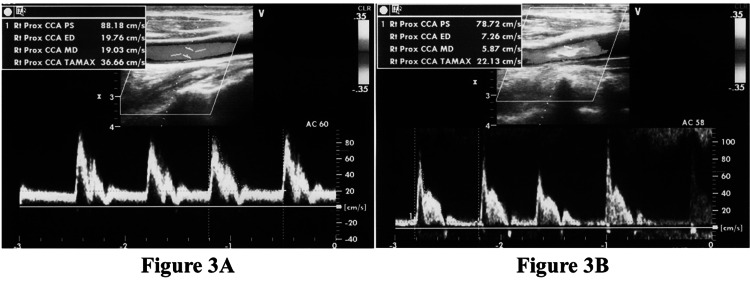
Ultrasound evaluation of a 73-year-old man with left hemiparesis. A: CCA waveform for the left carotid artery without a suspected occlusion. The ED velocity is 19.76 cm/s. B: CCA waveform for the right carotid artery with a suspected occlusion. Compared to the left side, the ED velocity is decreased to 7.26 cm/s. The calculated ED ratio is 2.72, and occlusion of right ICA or MCA is suspected. Abbreviations: CCA, common carotid artery; ED, end-diastolic; ICA, internal carotid artery; MCA, middle cerebral artery.
